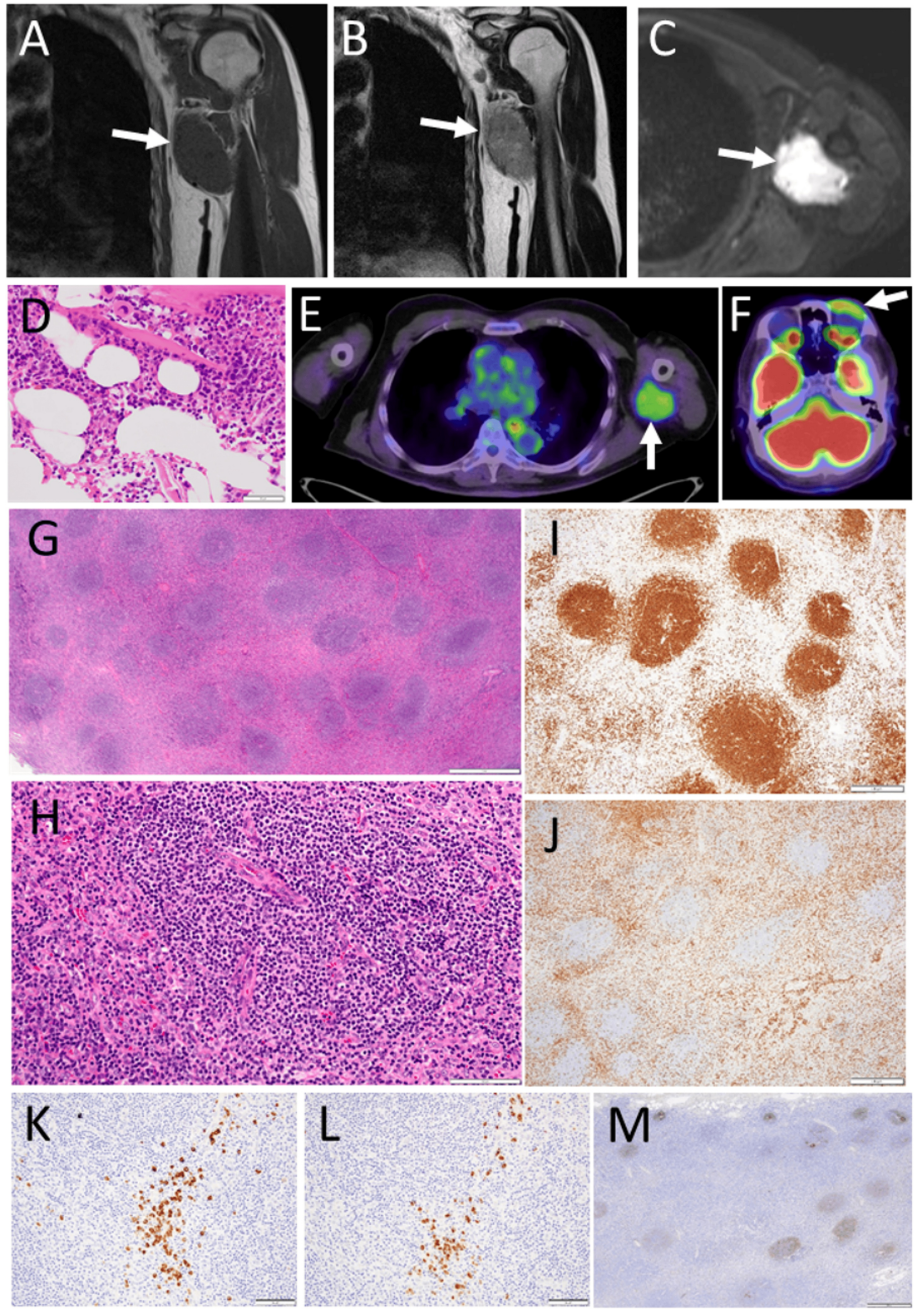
Left axillary mass. Magnetic resonance imaging at the age of 76 years, showing left axillary mass (arrows) in T1-weighted (A) and T2-weighted (B) coronal images and diffusion-weighted axial image (C). Iliac bone marrow biopsy (D), showing normocellular marrow with no anomalous cells. Positron emission tomography with axial computed tomography scans, showing high uptake (arrows) at left axillary mass with SUVmax at 3.51 (E) and in left superior anterior orbit (F). Incisional biopsy of left axillary mass, leading to the diagnosis of Castleman disease with hyaline vascular type at the age of 76 years. Hyperplasia of lymphoid follicles (G) with atrophic germinal center and dominant mantle layer. Prominent small vessels in follicular center (H). CD20-positive B-lymphocytes (I) and CD3-positive T-lymphocytes (J) segregate from each other. By in situ hybridization, immunoglobulin light chain κ (Igκ)-positive cells (K) and immunoglobulin light chain λ (Igλ)-positive cells (L) are equally distributed, indicating the bitype. CD21-positive follicular dendritic cells appear coarse (M). White scale bar = 50 µm in D, 1000 µm in G, 100 µm in H, K, L, 500 µm in I, J, M.
